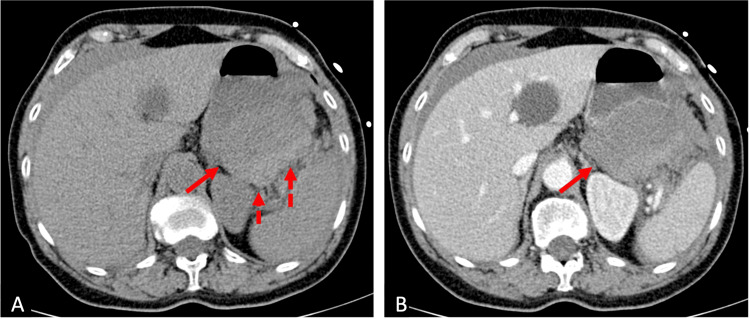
Non-contrast-enhanced (A) and contrast-enhanced (B) CT scan showing a voluminous hematoma (arrows) between the pancreatic tail and posterior stomach wall, with spontaneously hyperdense content in a dependent position (dashed arrows) indicating fresh blood.
